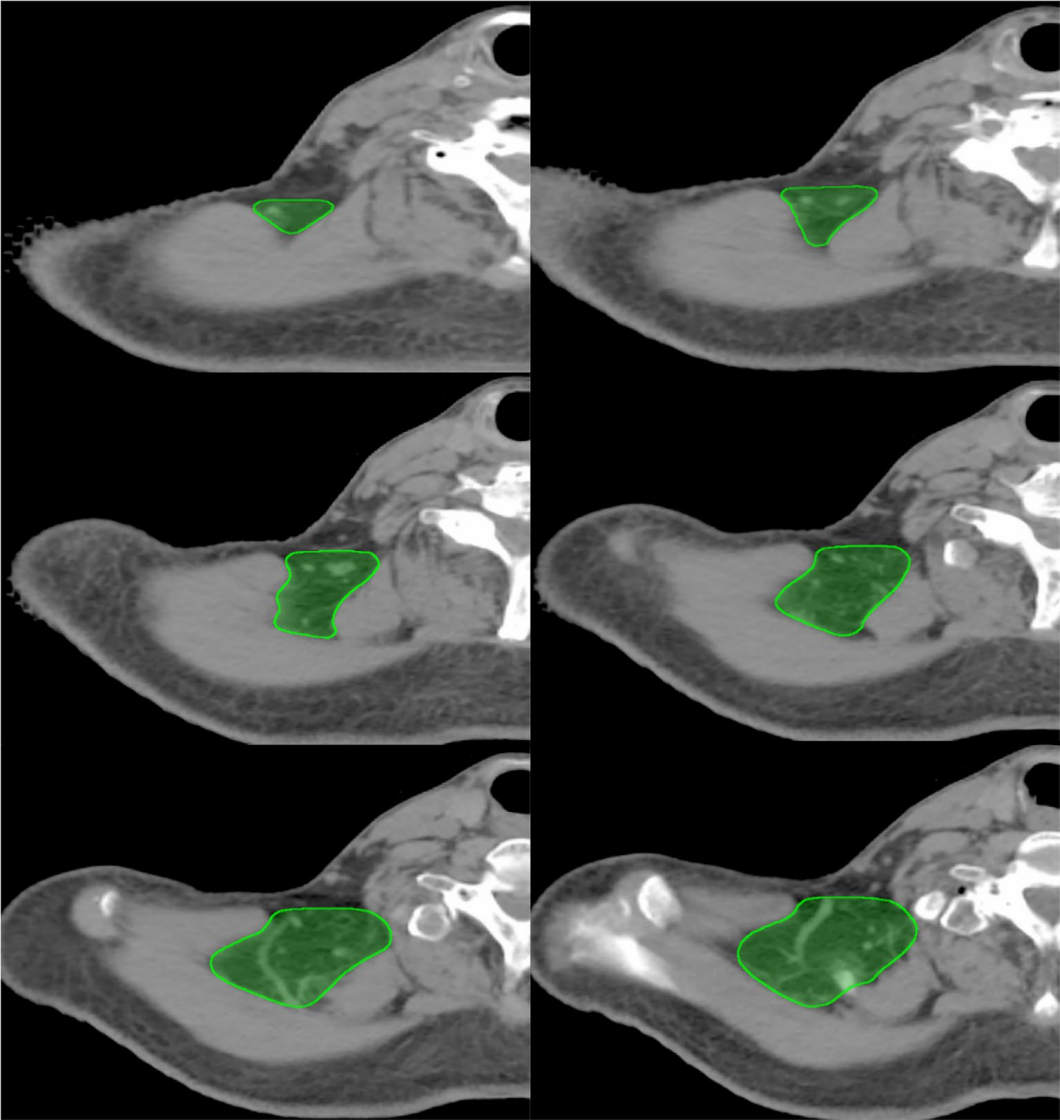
A set of CT scans to show how the PLV region is delineated, the green line represents the PLV region.
